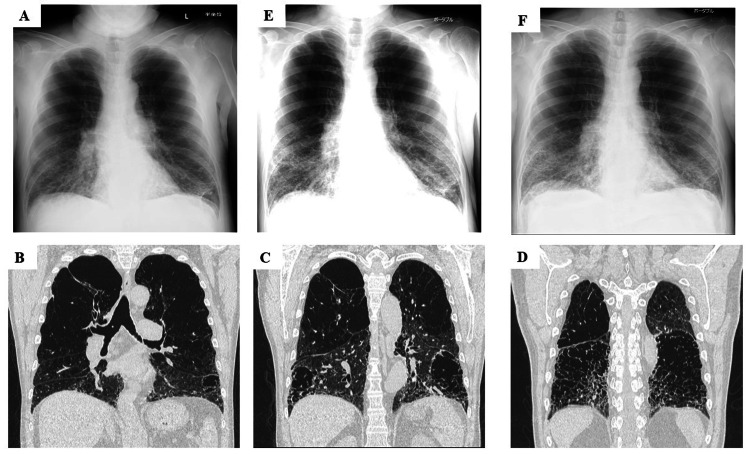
Chest radiographic images taken at different time points Chest X-ray (A) and computed tomography (B, C, and D) on admission show diffuse ground-glass shadows in bilateral lung fields. Diffuse ground-glass shadows on chest X-ray worsened by day 8 (E). Tocilizumab and baricitinib treatment improved findings on chest X-ray by day 25 (F).
